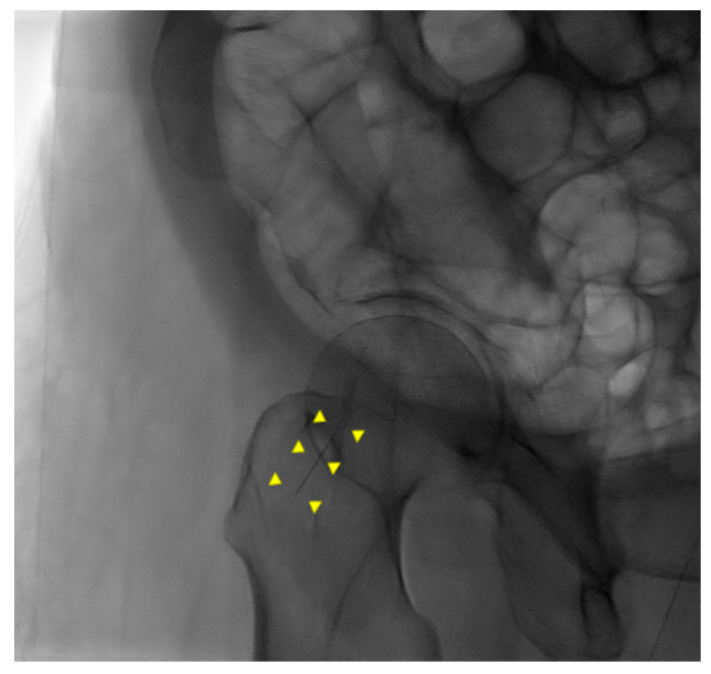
Fluoroscopic radiography of pelvic bone. A needle fragment showed in the right groin (yellow triangles).
